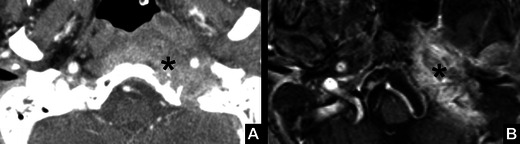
Osteomyelitis of the skull base.* A 74-year-old man presenting with slowly progressive symptoms of left hypoglossal nerve palsy and left VCP. CT and MR images at the level of the skull base (*not displayed*) showed fluid in the mastoid and middle ear bilaterally. a Contrast-enhanced CT and b) T1-weighted contrast-enhanced images with fat saturation showed infiltration of the area between the petrous apex and the temporo-mandibular joint by a destructive enhancing mass (*). The imaging findings suggested osteomyelitis and soft tissue infection. The jugular foramen and thereby the vagal nerve were obviously involved, causing VCP. The patient underwent left-sided exploration and mastoidectomy revealing massive infection of the middle ear, skull-base and neck
